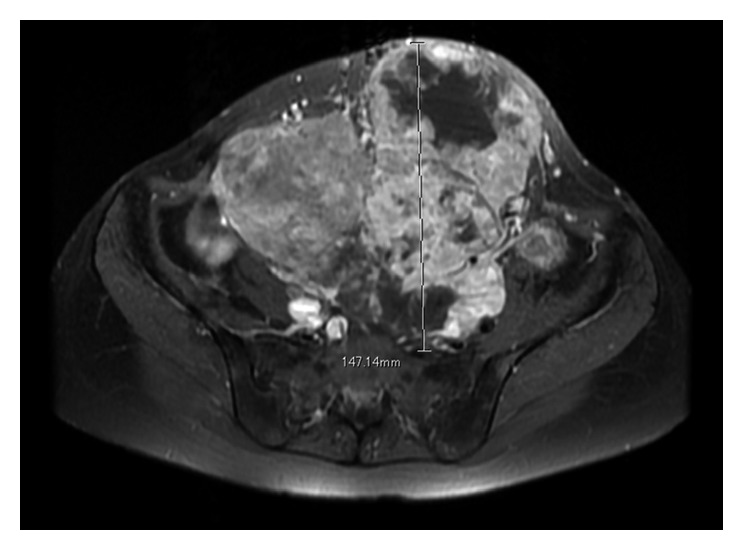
Magnetic resonance imaging findings (T1-weighted fat suppression gadolinium-enhanced image) after a drug holiday for six weeks. Tumor regrowth with increased enhancement was observed.
